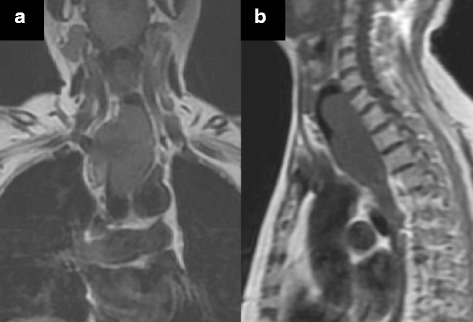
Magnetic resonance imaging. a, b MRI showed uniformity and clear boundary tumor
